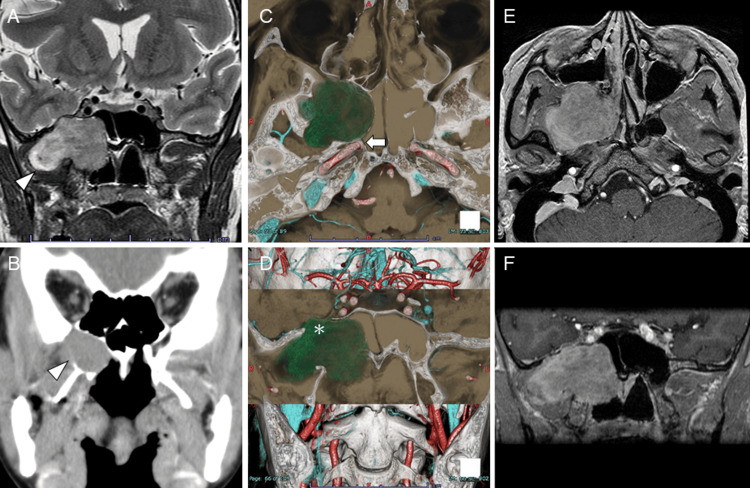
Preoperative CT and MRI images of the patient. (A) T2-weighted MRI reveals a mass lesion located on the right side of the pterygopalatine fossa. The lesion shows internal heterogeneity and moderate-to-high signal, extending into the inferior temporal fossa (arrowhead). (B) A tumor was incidentally visible in the right pterygopalatine fossa on a CT scan seven years previous, but it did not extend into the inferior temporal fossa (arrowhead). (C, D) Three-dimensional CT shows a well-defined, limbally smooth lobulated mass with a maximum size of 75 mm in the right pterygopalatine fossa to the inferior temporal fossa, with a dilated right median foramen (asterisk). The right internal carotid artery and mass are bordered by the bony wall of the carotid canal (arrow). (E, F) T1-weighted contrast-enhanced MRI shows evidence of enhancement of the mass.
